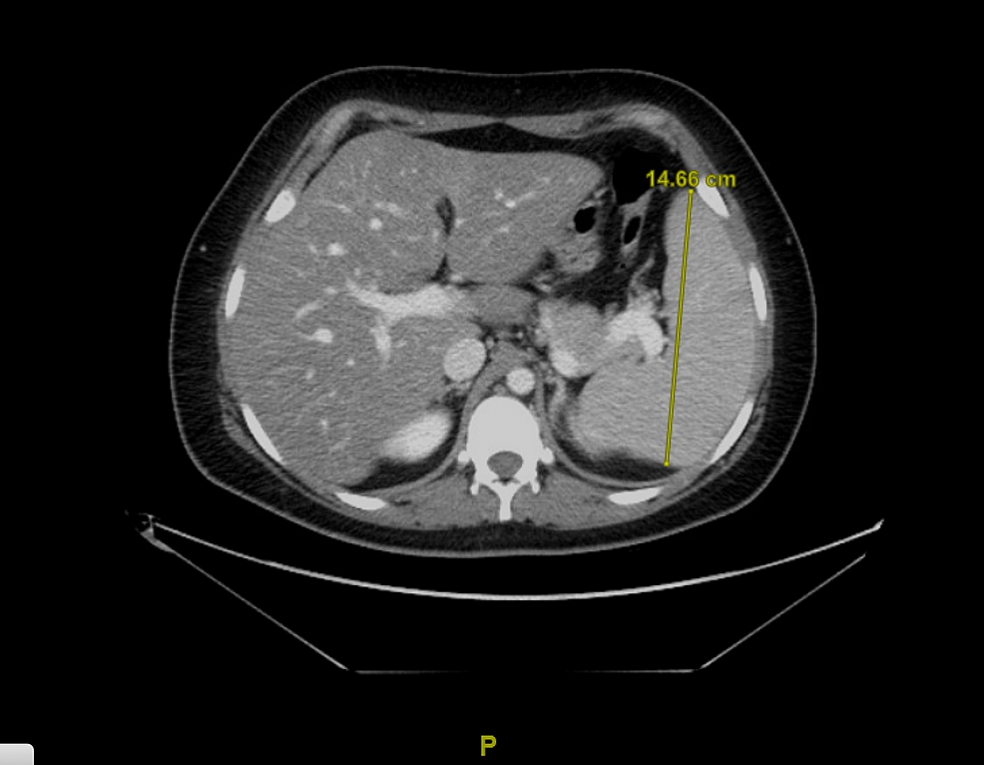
CT abdomen and pelvis with contrast showing splenomegaly measuring 14 cm CT: computed tomography
